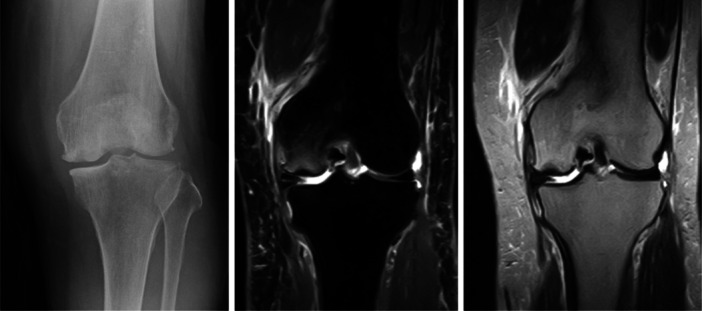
Stage 4 SIFK‐related osteonecrosis (or SONK) on plain radiographs and magnetic resonance imaging in an 85‐year‐old female patient. SIFK, subchondral insufficiency fracture. SONK, spontaneous osteonecrosis of the knee.
